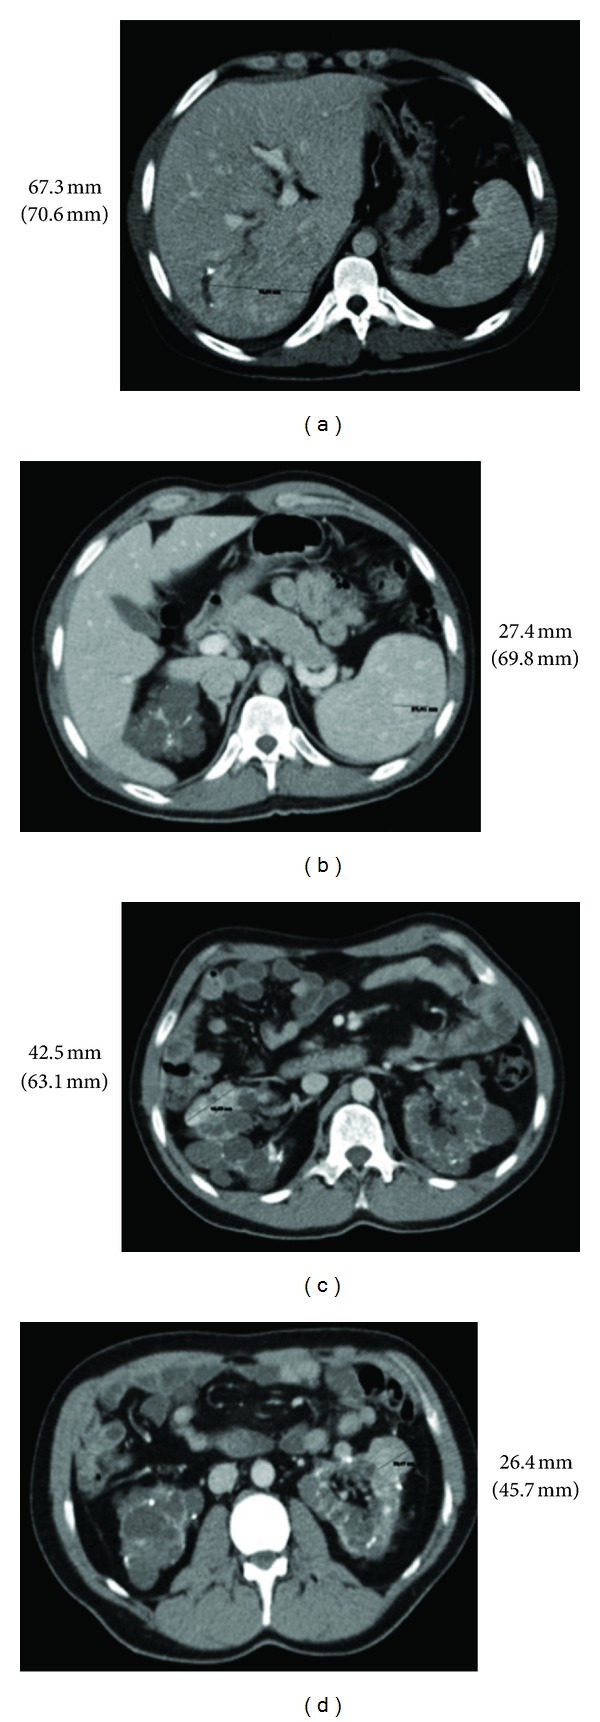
Abdominal CT performed after two years of treatment with rapamycin, showing a significant reduction in the size of tumours.
